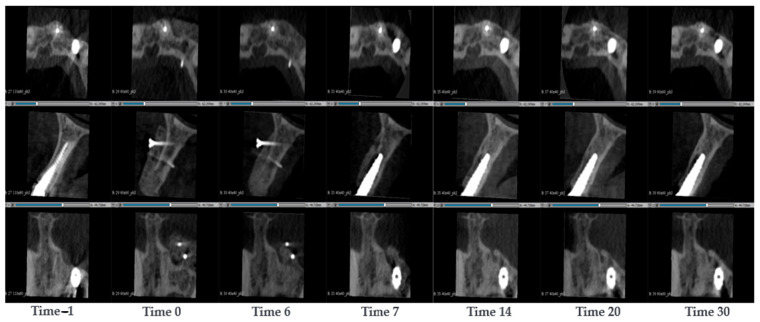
CBCT images of a 44-year-old woman treated for a thinning of the left maxillary, threatening the integrity of a dental implant. The implant was removed and a SmartBone^®^ plate was implanted to recover the maxillary thickness. The images show the progression of bone healing over time, from pre-surgery (Time −1) to 11 months post-surgery (Time 30).
